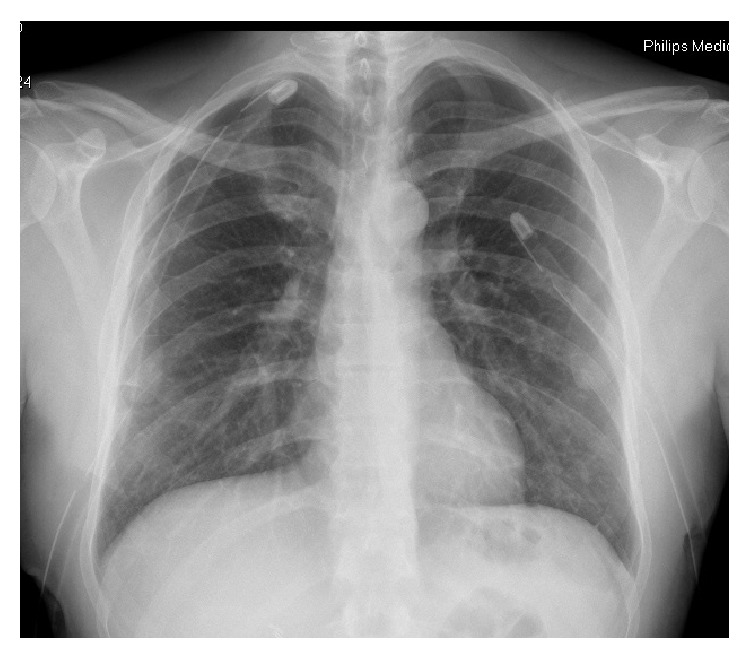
Chest X-ray showing reexpansion of the lungs following bilateral chest tube insertion.
